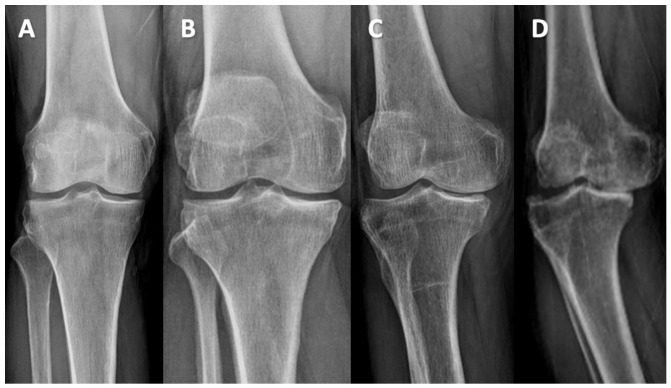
Classification of patients with knee osteoarthritis according to K-L stages. Stage 1 (A), Stage 2 (B), Stage 3 (C), and Stage 4 (D) patients’ anteroposterior knee joint X-ray images.
